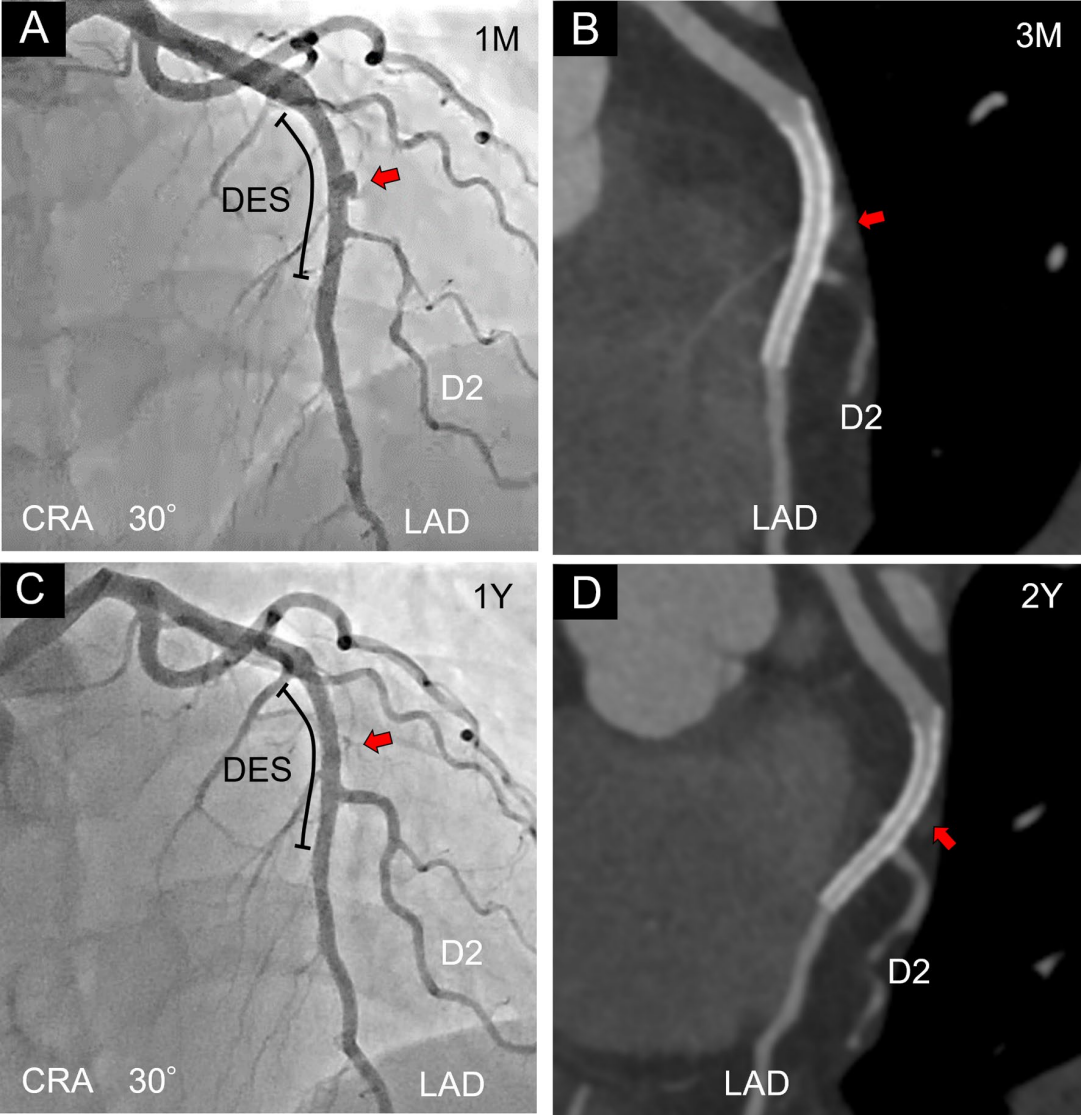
Follow-up of the AFL after PCI. A Coronary angiography (CAG) (CRA 30°) at 1 month after percutaneous coronary intervention (PCI) still demonstrated contrast accumulation outside the drug-eluting stent (DES) (red arrow). B Coronary computed tomography (CT) angiography showed decrease in size of the aneurysmal false lumen (AFL) at 3 months after PCI (red arrow). C One year after PCI, CAG (CRA 30°) showed the disappearance of the AFL (red arrow). D Coronary CT angiography revealed no contrast accumulation outside the DES (red arrow) and showed no restenosis inside it at 2 years after PCI. CRA: Cranial, DES: Drug-eluting stent, D2: Second diagonal branch, LAD: Left anterior descending artery, 1 M: 1 month, 3 M: 3 months, 1Y: 1 year, 2Y: 2 years
